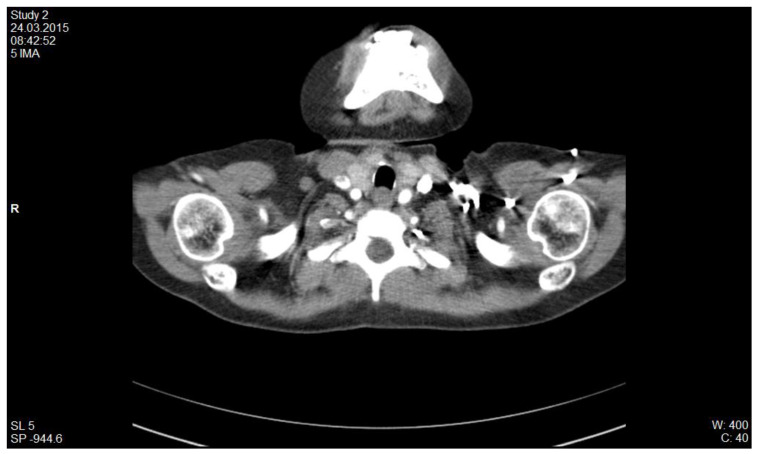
CT scan, right IJV thrombosis, calcifications in the trachea in the context of calciphylaxis.
